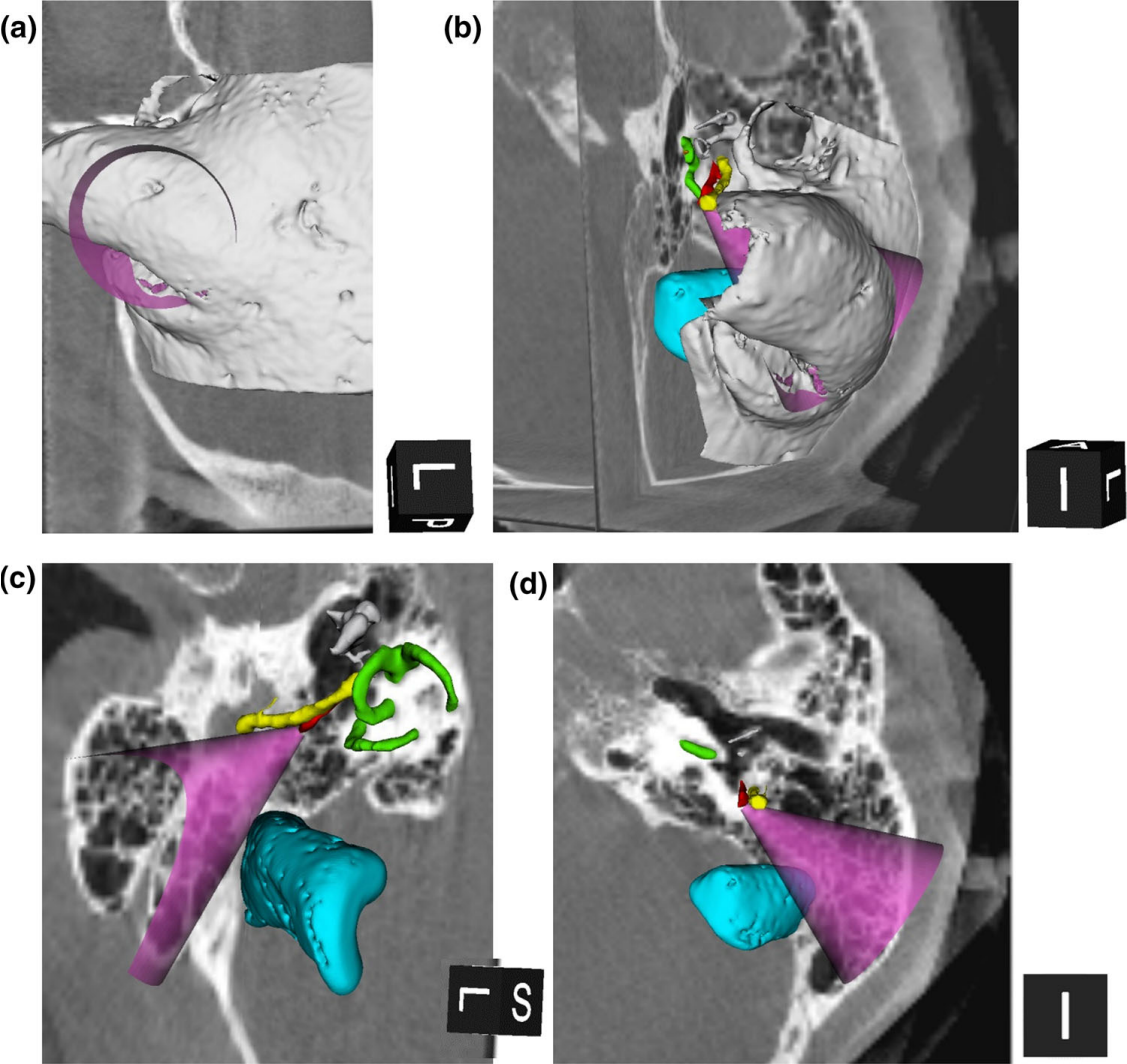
The automatically generated cone-shaped surgical corridor (purple) is superposed onto the 3D reconstruction and Dyna-CT axial and sagittal slices. a and b show the temporal bone surface, whereas c and d show the corresponding inner structures, with the cone-shaped surgical corridor (purple) positioned with the tip on the target center. The cubes at the bottom right of each picture give the orientation: L = lateral; P = posterior; I = inferior
